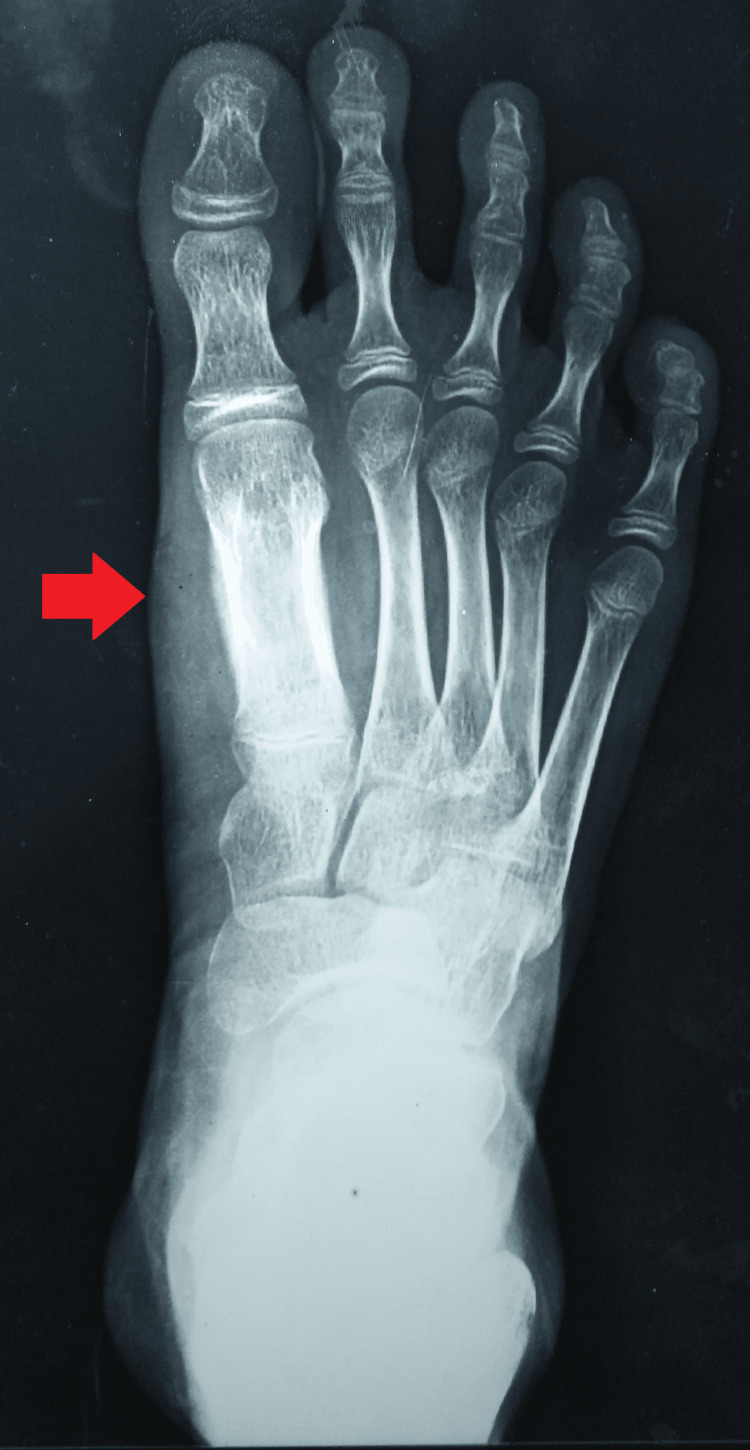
Plain radiograph (A-P) view of the right foot showing soft tissue swelling and involvement of the first metatarsal head A-P: antero-posterior
